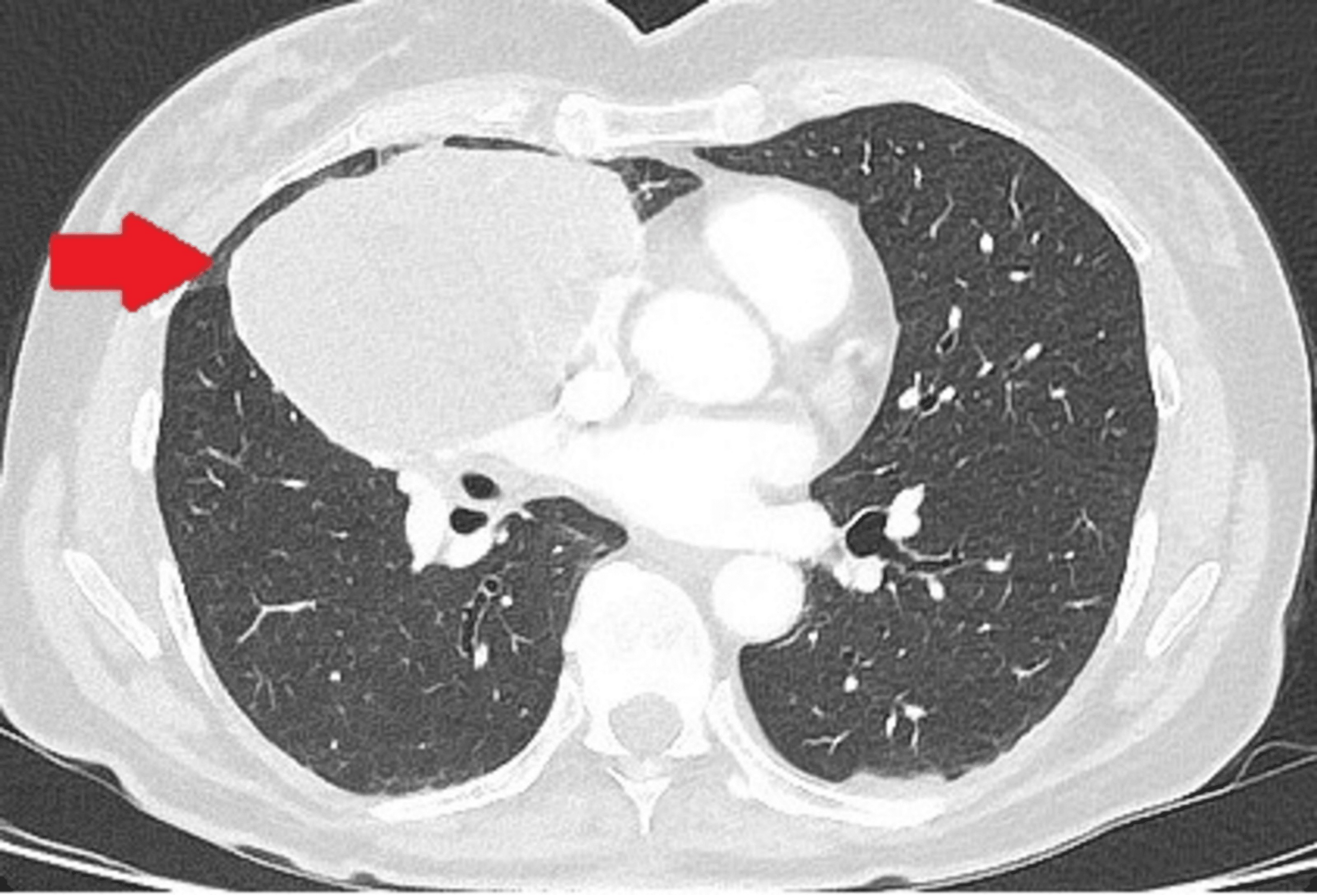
Preoperative CT scan The cross-sectional view of the CT scan shows a large mass in the right chest.
